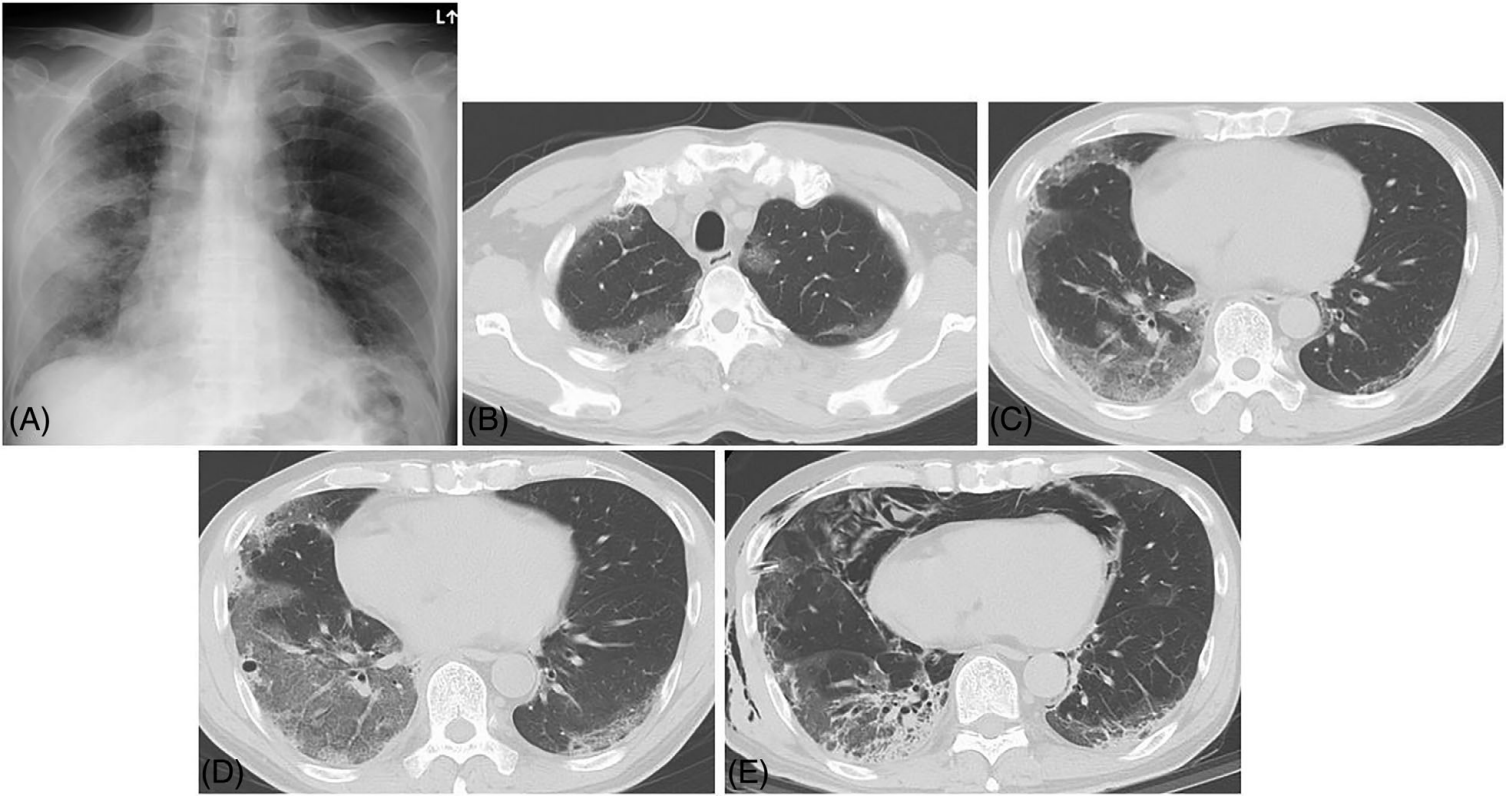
Radiological findings. (A) Chest x‐ray showing bilateral ground‐glass opacity (GGO) which was dominant in the right lung. (B, C) High‐resolution computed tomography (HRCT) on day 1. The area of the perilobular GGO in the upper lobes and lower lobes. (D) HRCT on day 5. The area of the GGO that had spread, and the cavity after cryobiopsy. (E) HRCT on day 23. Radiological fibrosis was remarkable on the lower lobe
